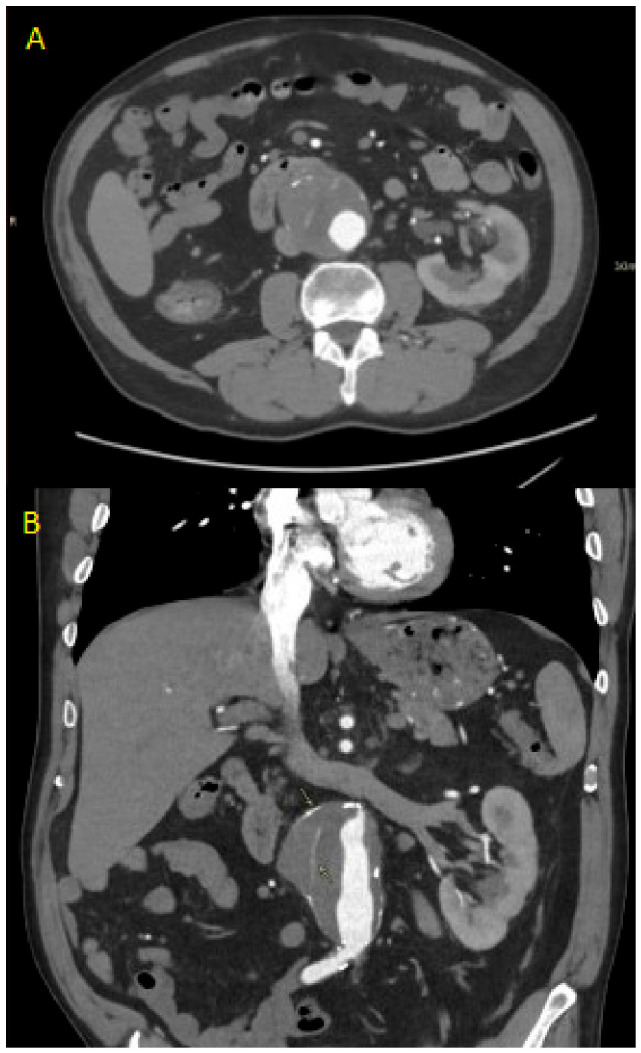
CT angiography ((A); axial; (B): coronal) showing a complex abdominal aortic aneurysm with interval growth, eccentric mural thrombus, and a residual intimal flap consistent with chronic dissection ((B), yellow arrows). These findings represent imaging markers of progression and wall instability, highlighting the role of CTA in acute evaluation and management planning.
